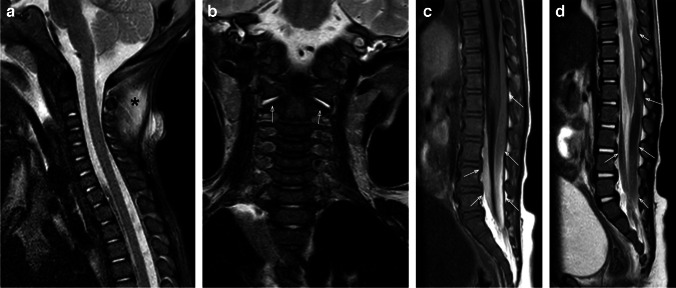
Fourteen-month-old boy presenting with lethargy and bruising. Sagittal STIR MR image (a) of the cervical spine demonstrates the nuchal ligament edema (*asterisk*) and dorsal soft tissue edema. Coronal T2-weighted MR image (b) shows fluid in both atlanto-axial joints (*arrows*). Sagittal T1-weighted (c) and T2-weighted (d) MR images of the lower spine show the T1-hyperintense and T2-hypointense subdural hemorrhage (*arrows*) extending inferiorly to the distal thecal sac
